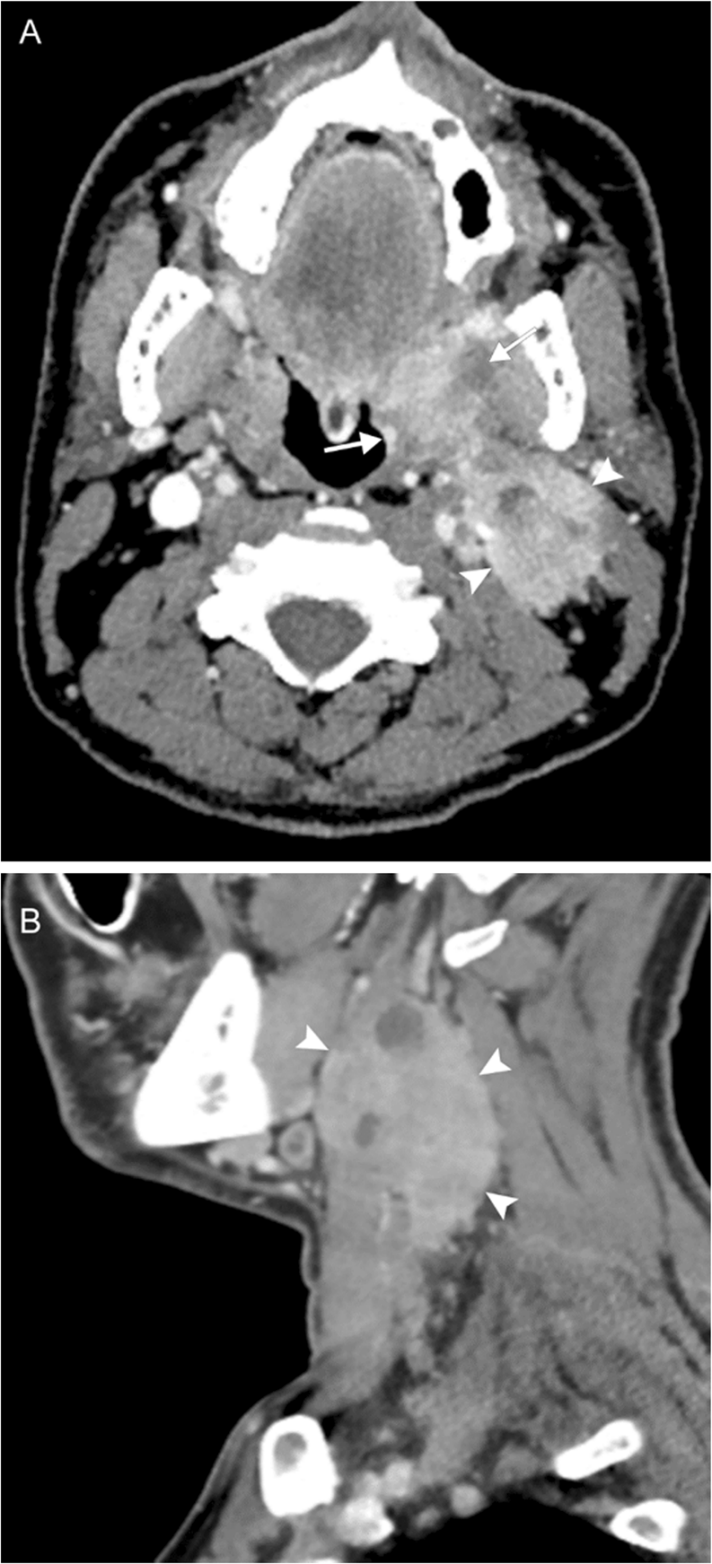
Oropharyngeal squamous cell carcinoma. A 40-year-old man presenting with tender neck mass, dysphagia to solid food, odynophagia, and voice changes. Axial (a) and sagittal (b) contrast-enhanced CT images reveal an enhancing mass at the left oropharynx (arrows) and extensive left-sided lymphadenopathy, including a large level II nodal conglomerate with central areas of necrosis (arrowheads). Biopsy revealed this to be human papilloma virus (HPV)-mediated squamous cell carcinoma. Voice change was attributed to encroachment upon the carotid space resulting in vagal nerve irritation.
